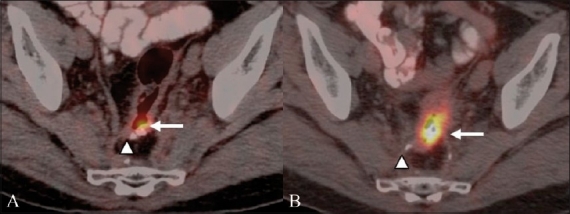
A 67-year-old man operated for rectosigmoid malignancy presented with rising tumor marker levels and underwent an FDG PET / CT study. The fusion FDG PET / CT image (A) reveals a tiny, but intense focus of FDG uptake (arrow) at the rectosigmoid anastomotic site. A contrast-enhanced CT scan of the abdomen and pelvis and colonoscopy, however, did not reveal recurrence; hence, the patient was kept under observation. A follow-up PET / CT study done after eight weeks (B) shows disease progression, by demonstrating increase in the extent and intensity of the FDG uptake, with the appearance of a soft tissue mass at the anastomotic site. A colonoscopic biopsy confirmed recurrence. Hyperdense surgical staples (arrowheads in A and B) mark the anastomotic site.
